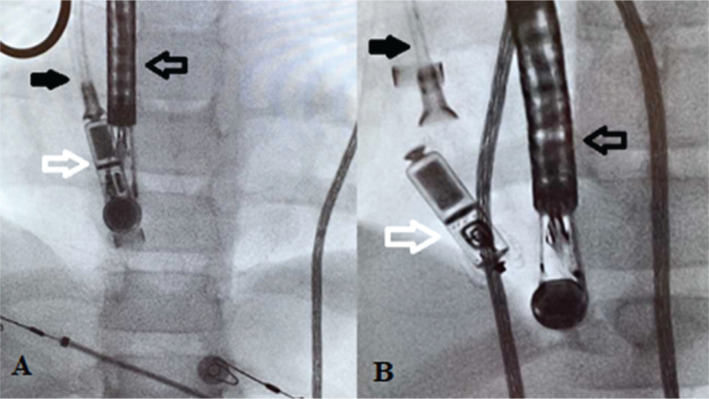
Fluoroscopy positioning demonstrating the final position of the Micra™ device. A: The Micra™ TPS was attached to the delivery cable, appropriately positioned with the cup attached to the mid-right interventricular septum. B: The device was deployed from the delivery cable and successfully implanted into the mid-RV septum under fluoroscopic guidance. Filled arrow: delivery cable; hollow black arrow: transesophageal echo probe; hollow white arrow: Micra™ TPS.
